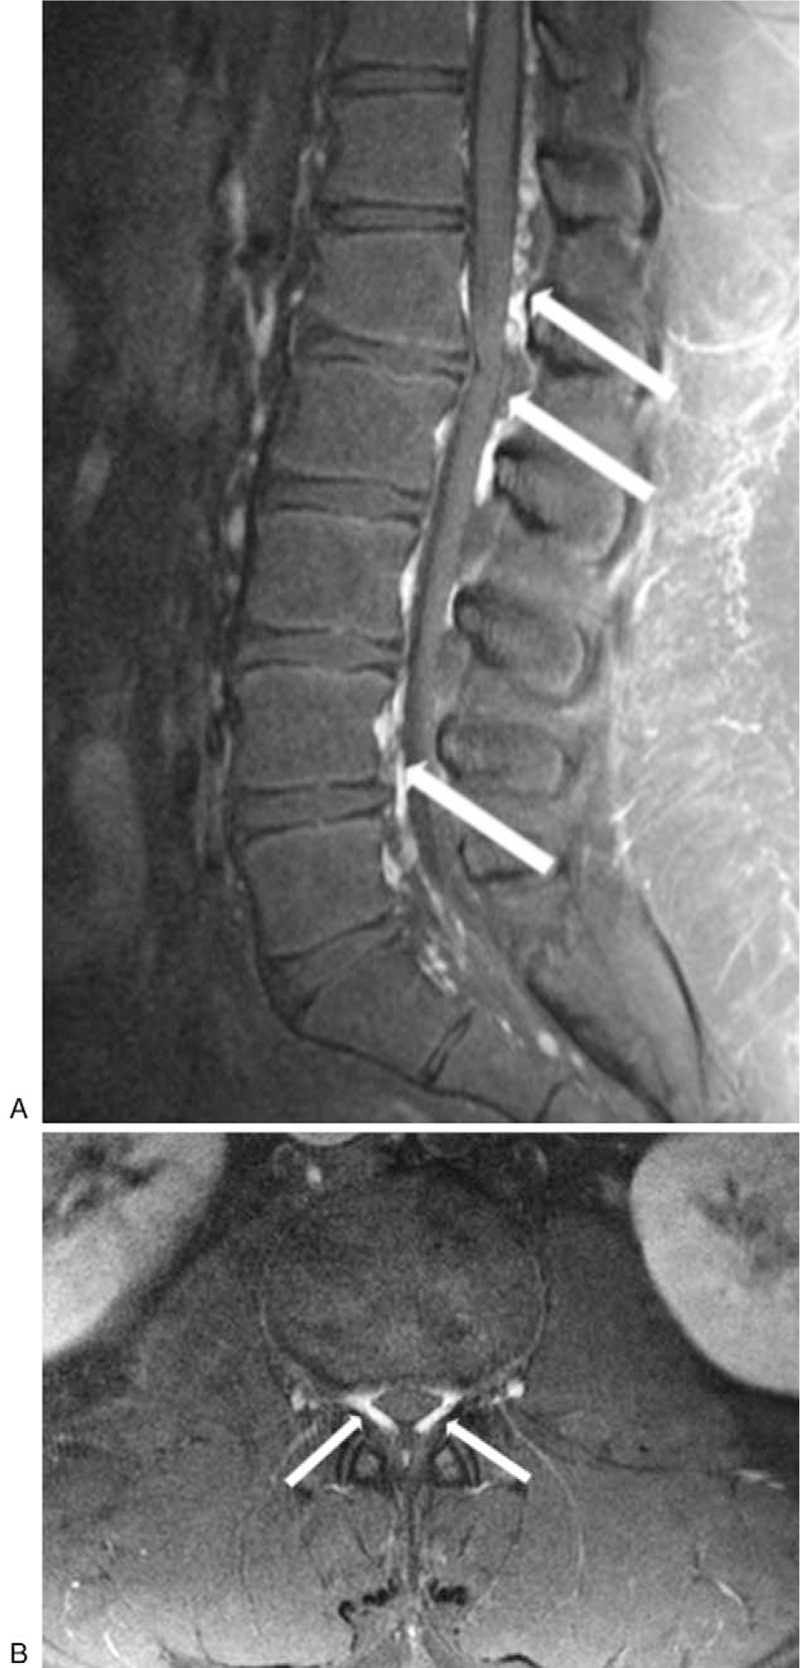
T2-weighted sagittal (A) and axial (B) magnetic resonance images show enlarged lumbar epidural veins (arrow) compressing the dural sac and causing disc herniation at the left L1–L2 level.
